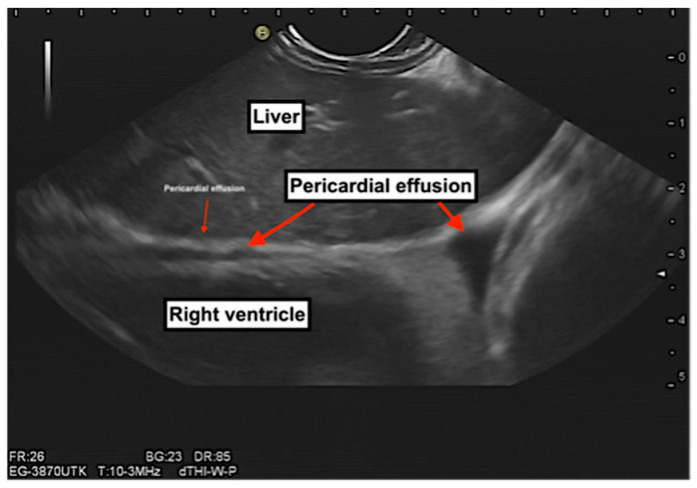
Endosonographic ultrasound demonstrated a minimal pericardial effusion.
